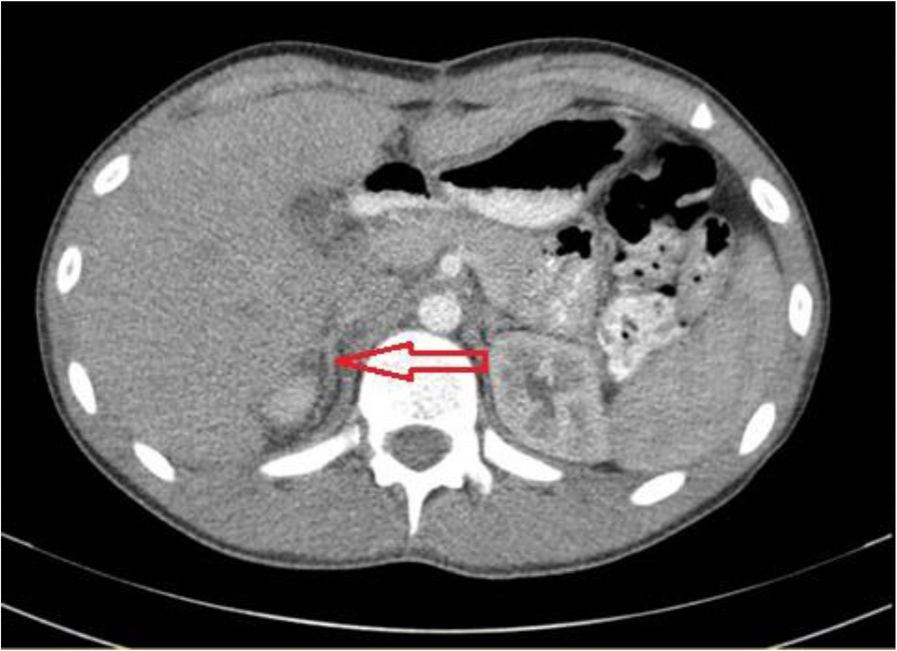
Normal appearance of adrenal glands is evident in postoperation computed tomography scan shown with *arrow* (after tumor resection and before adrenalectomy)
